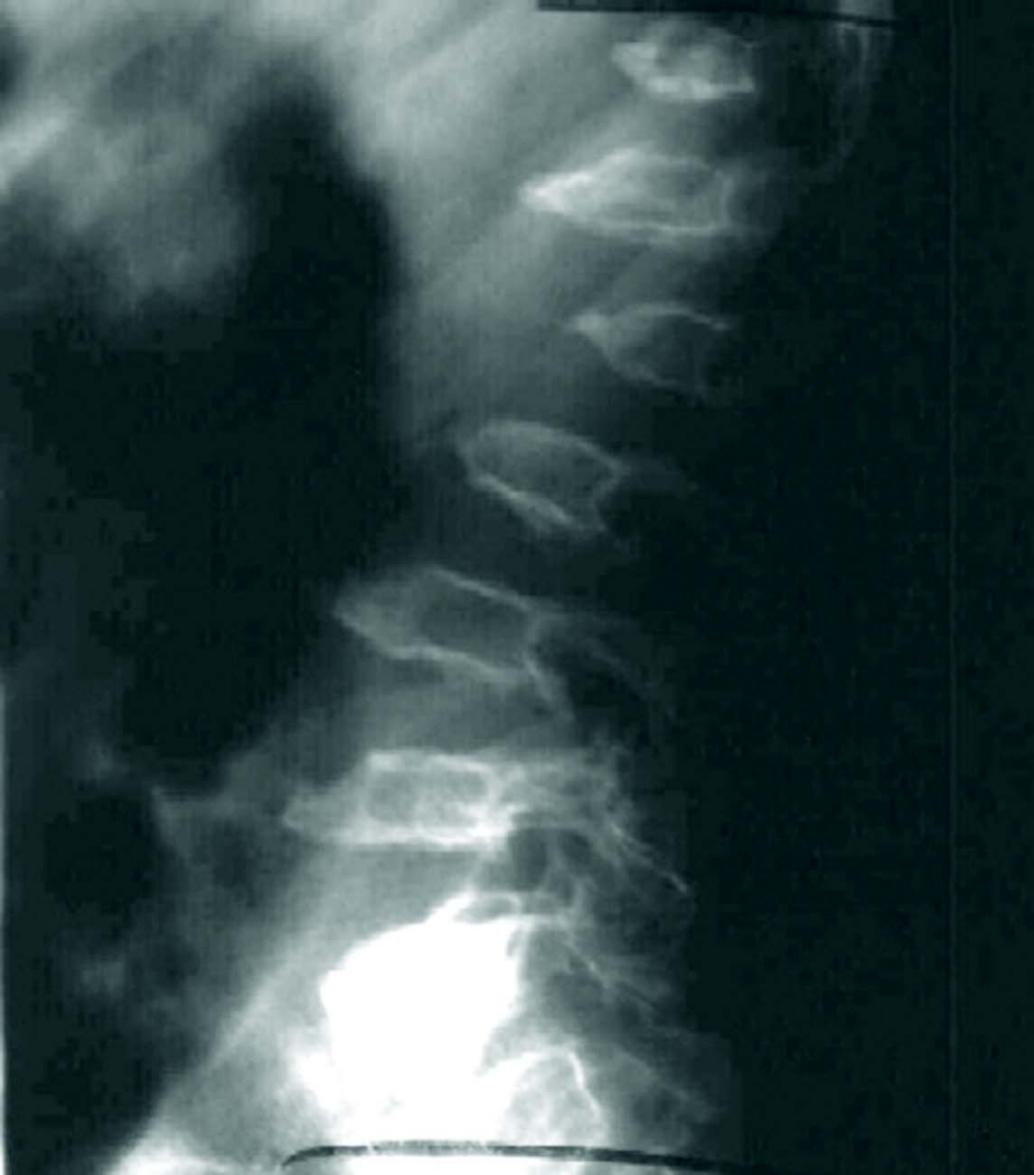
Rapidly progressing 8-year old male MPS VI patient: radiograph of spinal column showing abnormal development of vertebral bodies, paddle-shaped widened ribs and short, thick irregular clavicles, lordosis, kyphosis and scoliosis.
